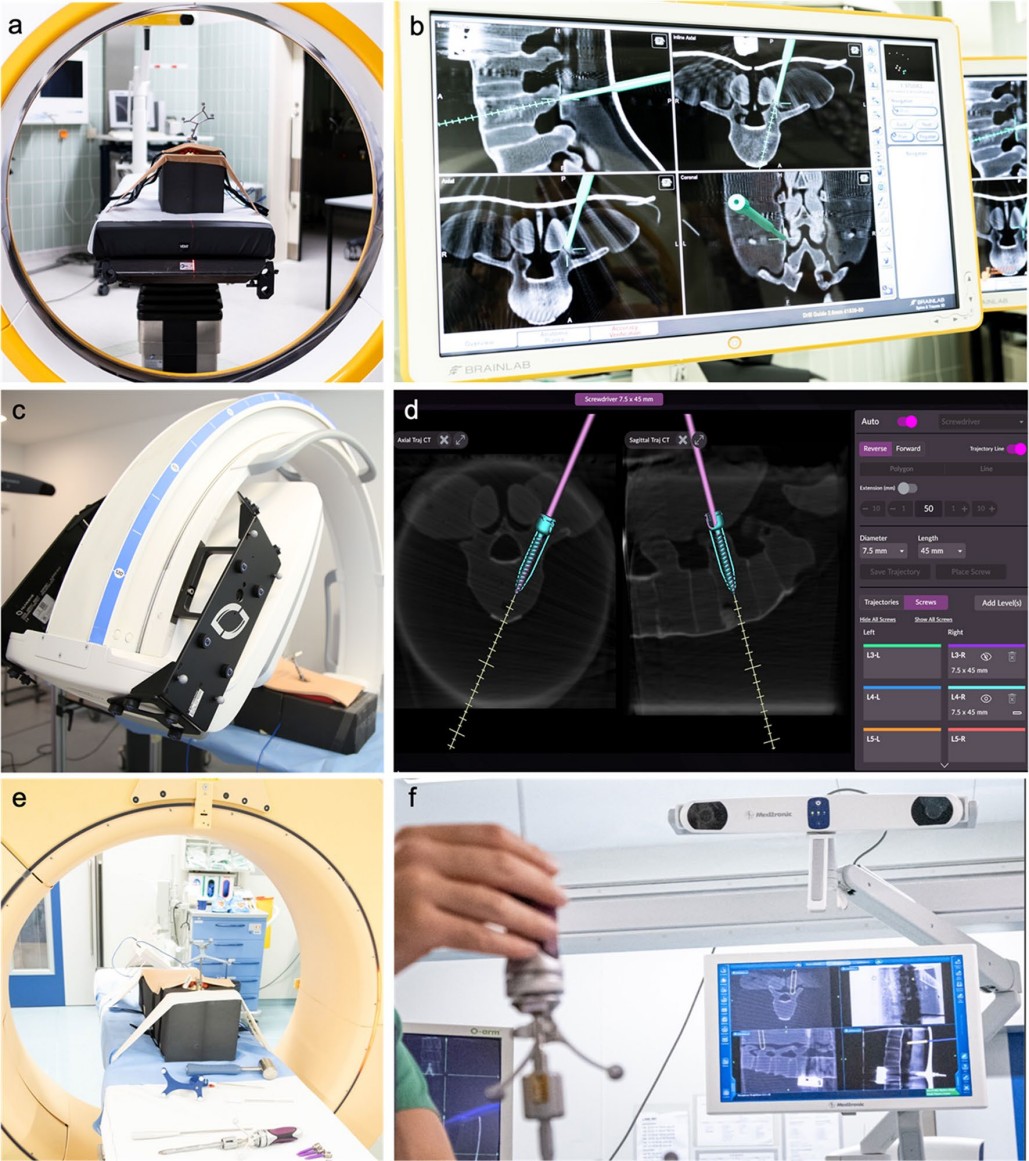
The images devices and respective navigation interfaces used in the study: iCT (a) with curve navigation (b), C-arm CBCT (c) with pulse navigation (d) and CBCT (e) with StealthStation navigation (f).
